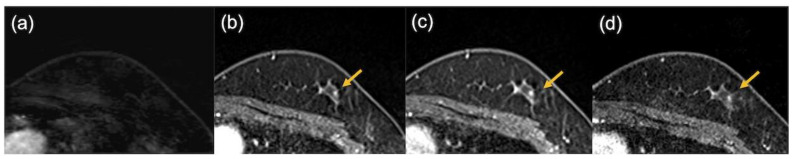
The images of a patient with triple-negative breast cancer after neoadjuvant chemotherapy. Pathological evaluation was complete response. No enhancing area was detected on the ultrafast dynamic contrast-enhanced MR image (a), while enhancing foci were observed in the early phase (b), the delayed phase (c), and the high spatial-resolution (d) images (arrows).
